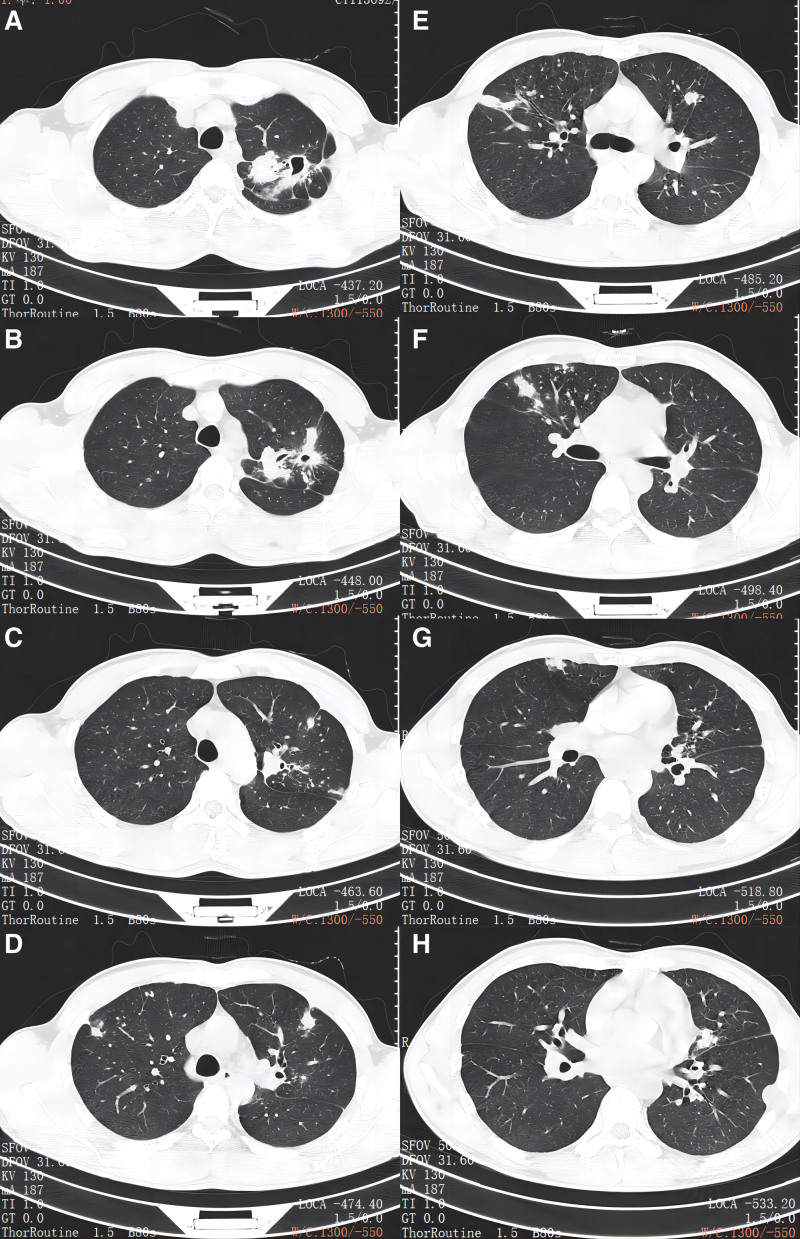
Chest CT of the patient taken on August 29, 2022.
